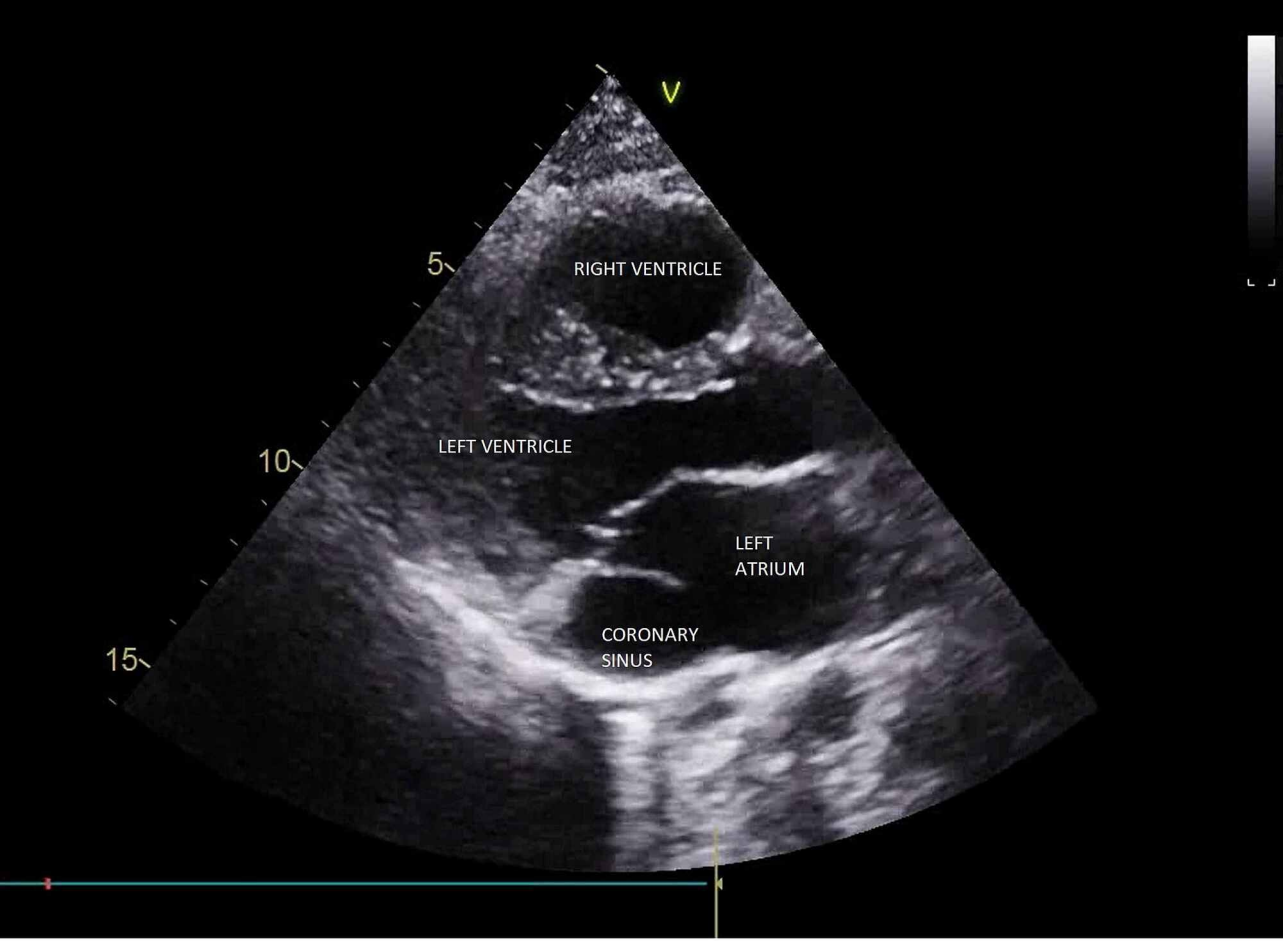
Parasternal long-axis view showing a dilated coronary sinus
